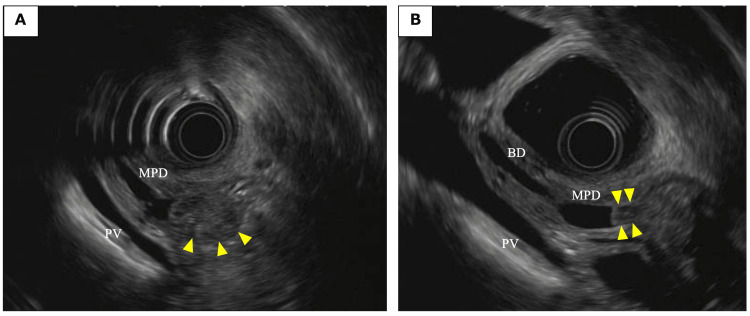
Findings of endoscopic ultrasound (EUS). (A) A hypoechoic tumor was found in the ampulla of Vater (arrows). (B) Infiltration of the main pancreatic duct (MPD) near the ampulla of Vater was suspected (arrows). BD: Bile duct; PV: portal vein
